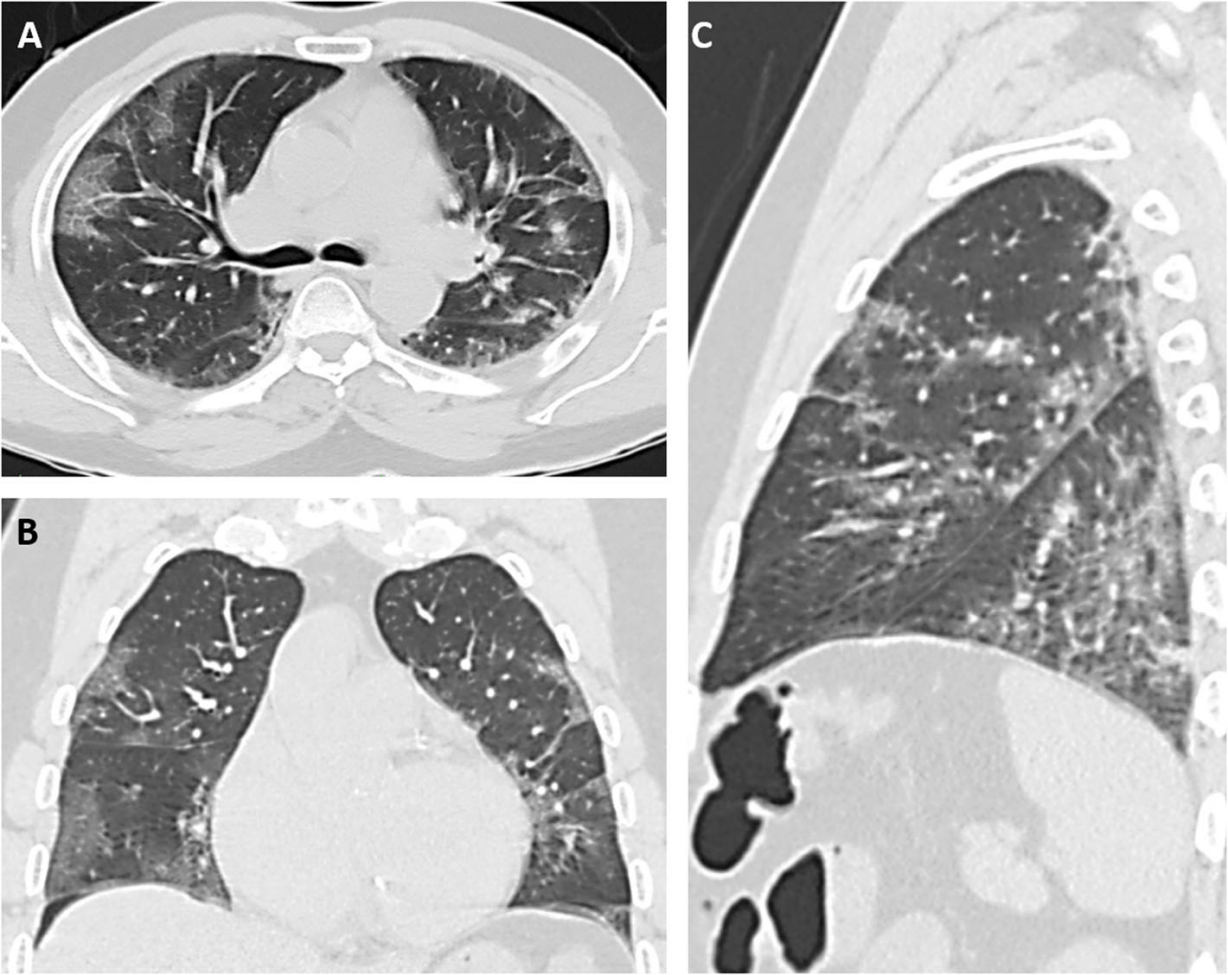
Non-contrast CT of a 42-year-old male with fever, sore throat, and shortness of breath, rt RT-PCR +VE, (A-C): A, axial; B, coronal; and C, sagittal non-contrast CT scan lung window show bilateral multifocal peripheral and pleural-based ground glass opacity with linear opacities and interlobular thickening
